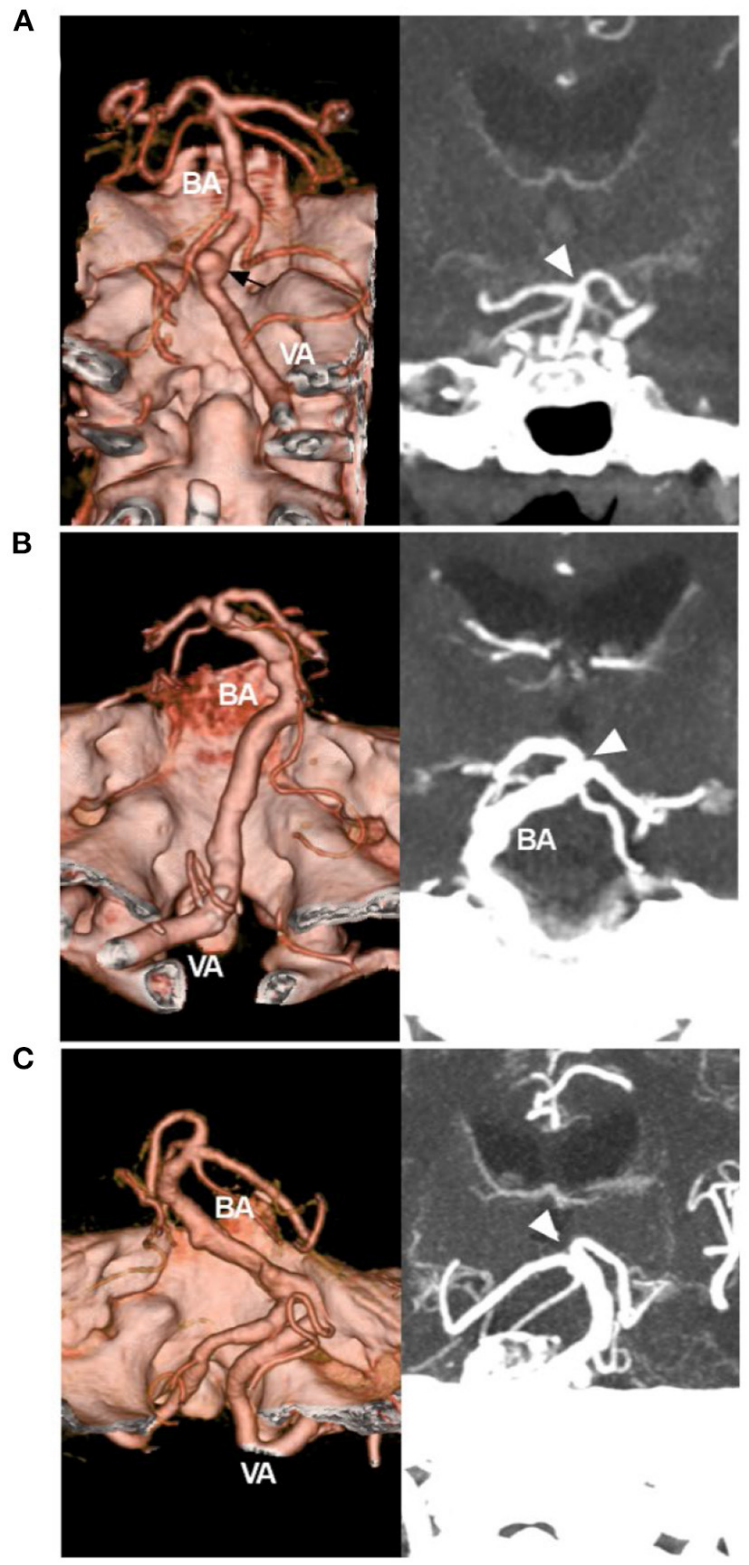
Simplified classification of VBD on CTA. (A) Left: CTA reconstruction image showing a mild VBD. The VBD did not reach the lateral margin of the clivus or dorsum sellae, and the arrow indicated an aneurysm. Right: MIP image showing that the BA tip (arrowhead) reached the floor of the third ventricle. The score of the Smoker et al. grading system was 3 (1 + 2). (B) Left: CTA reconstruction image showing a moderate VBD; the VBD reached the lateral margin of the clivus or dorsum sellae; right: MIP image showing that the BA tip (arrowhead) elevated the floor of the third ventricle; the score was 5 (2 + 3). (C) Left: CTA reconstruction image showing a severe VBD, the VBD reached the cerebellopontine angle cistern; right: MIP image showing the BA tip (arrowhead) elevating the floor of the third ventricle; the score was 6 (3 + 3). BA, basilar artery; CTA, computed tomography angiography; MIP, maximum intensity projection; VA, vertebral artery; VBD, vertebrobasilar dolichoectasia.
